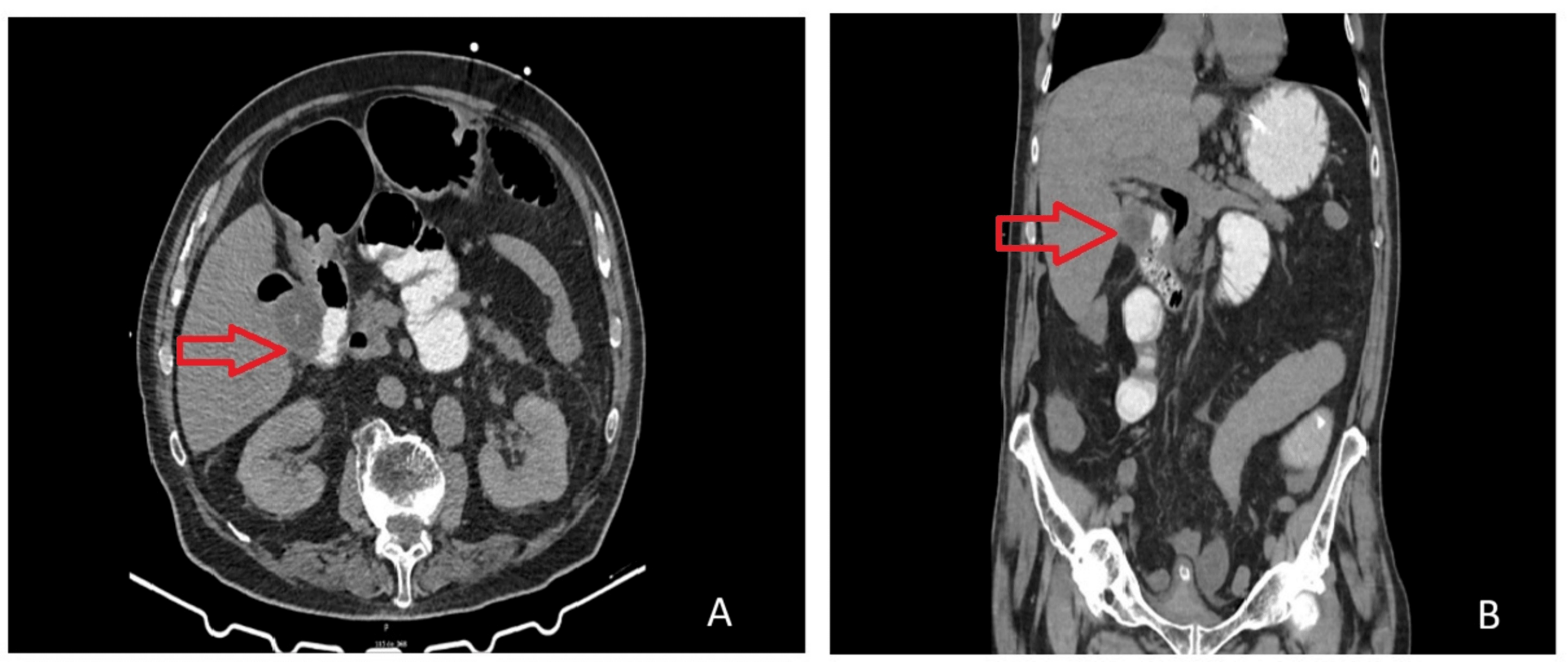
Axial and coronal section of simple abdominal CT Repeat CT shows diffuse dilation of the small bowel  with a cholecystoenteric fistula between the gallbladder fundus and the second duodenal portion (red arrow)
